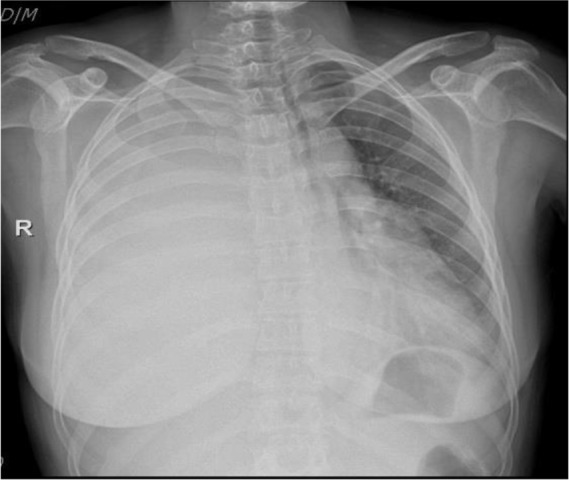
Chest X-Ray radiograph shows right side white lung and mediastinal shifting to the left
